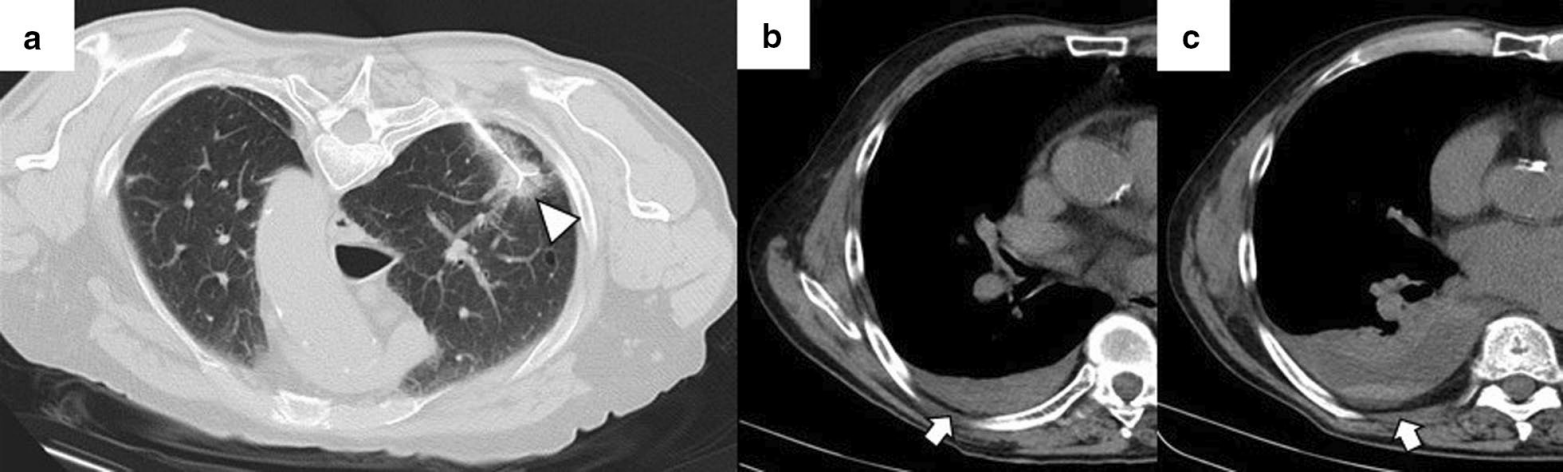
Mild hemothorax after image-guided percutaneous lung ablation. a CT-guided percutaneous RFA of a stage 1 NSCLC located in the lower left lobe. Note the expanding tube encompassing the entire lesion. b CT scan immediately after the procedure where we observe a mild hyperdense pleural effusion, consistent with a mild hemothorax. c CT scan performed 24 h after the procedure shows a slight increase in the hyperdense pleural effusion and a discrete hyperdense level within the pleural effusion, confirming the hemothorax (arrow). However, after 48 h of clinical and radiological stability, the patient was discharged
